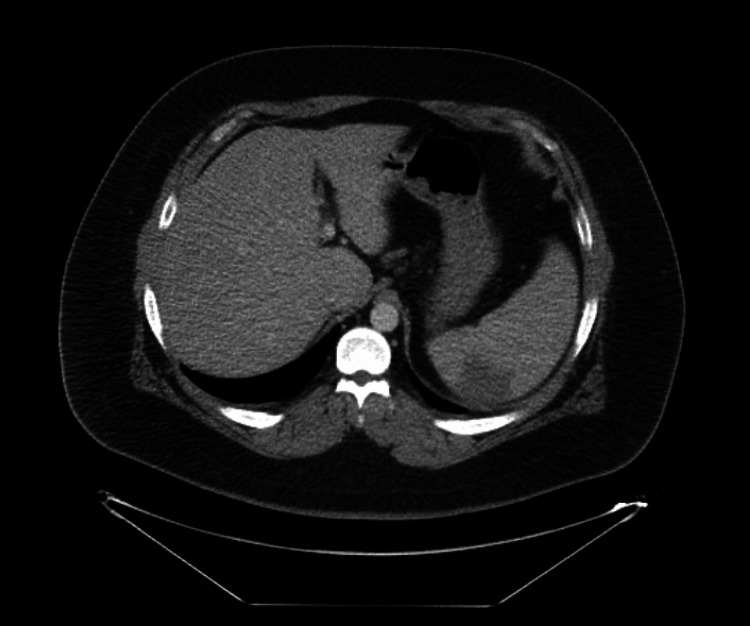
CT abdomen and pelvis with IV contrast (axial view). Triple-phase abdominal CT reveals wedge-shaped hypodensity at the posterior and medial aspects of the spleen. CT, computed tomography; IV, intravenous
